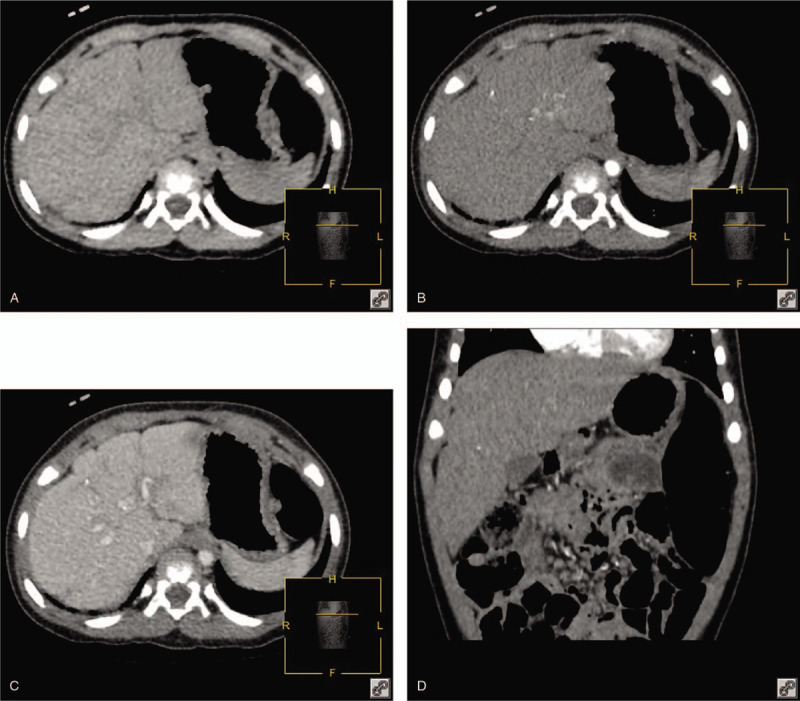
One year later, computed tomography scan of the abdomen showed the liver volume and size of the lesions had decreased considerably.
